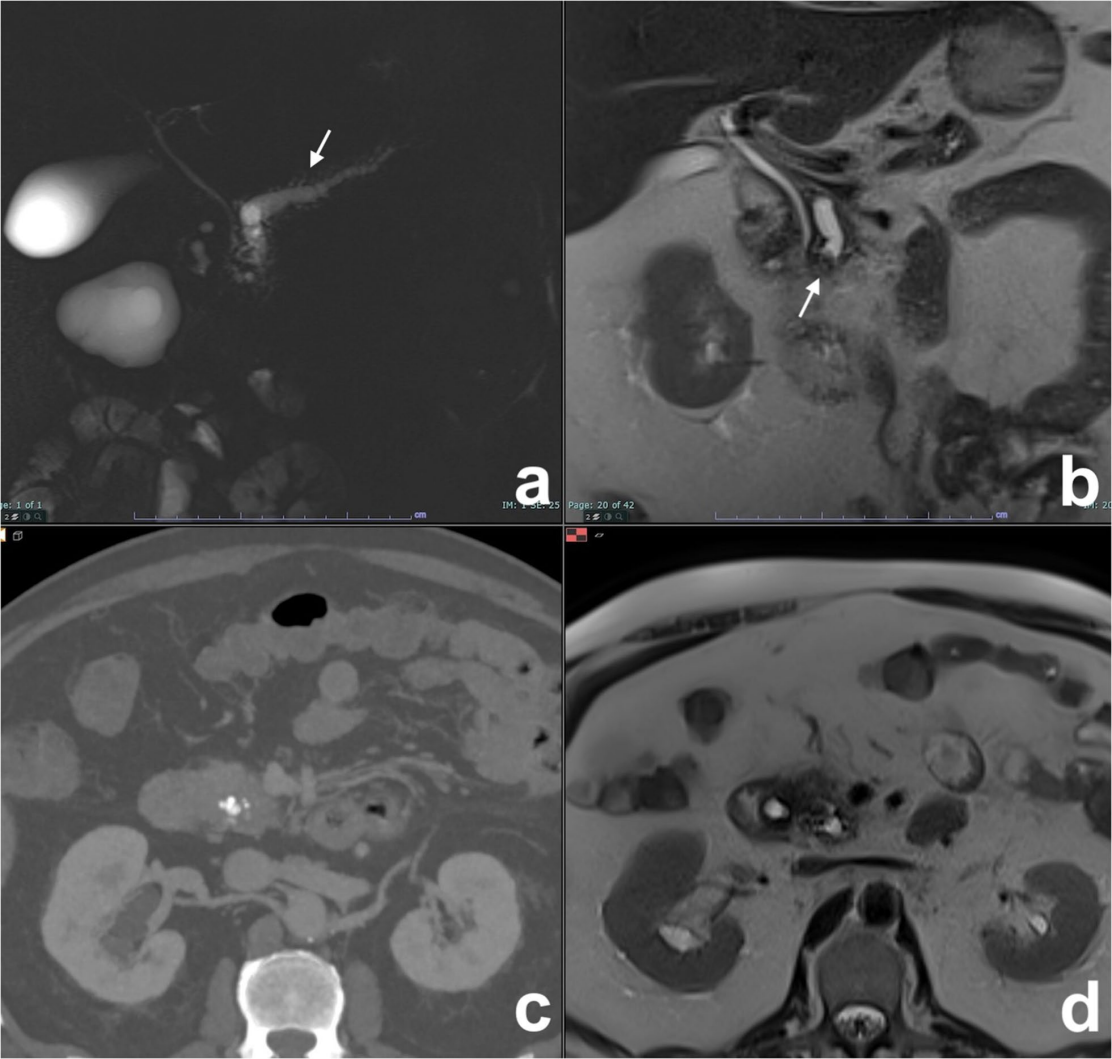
Chronic obstructive pancreatitis. At MRCP (a), a diffuse dilatation of the main pancreatic duct is appreciable through the gland with finger-like dilatation of branch ducts (arrow). At T2w, a defect in the prepapillary portion of the wirsung duct is appreciable (arrow). At unenhanced CT (c), a gross calcification is visible in the head of the pancreas, related to the intraductal calculus, responsible for the dilatation of the wirsung duct upstream, not visible on axial T2w (d)
